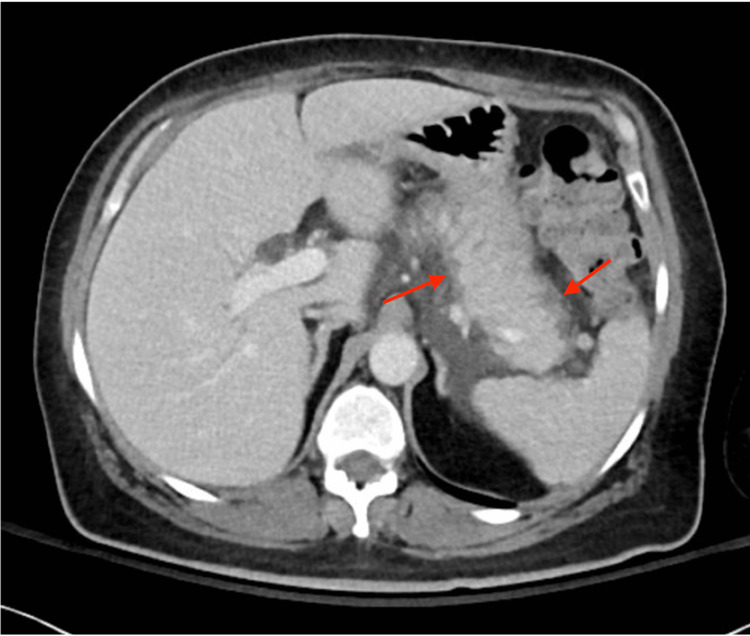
Axial abdominal CT showing diffuse enlargement of the pancreatic body and tail with peripancreatic fat stranding and no evidence of pancreatic necrosis Red arrows indicate peripancreatic fat stranding, a hallmark radiologic feature of acute pancreatitis, reflecting inflammation extending into the surrounding fat.
